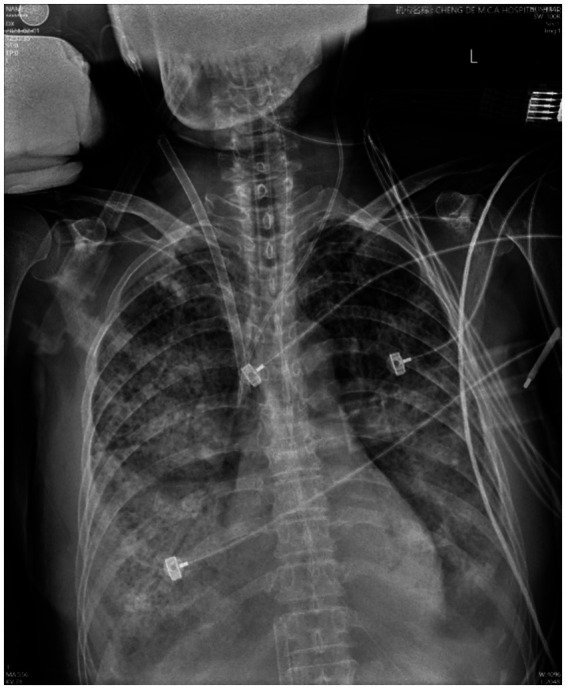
Chest X-ray images of the patient. The chest X-ray image, obtained on day 2, showed multiple patchy and flaky shadows in the lungs.
